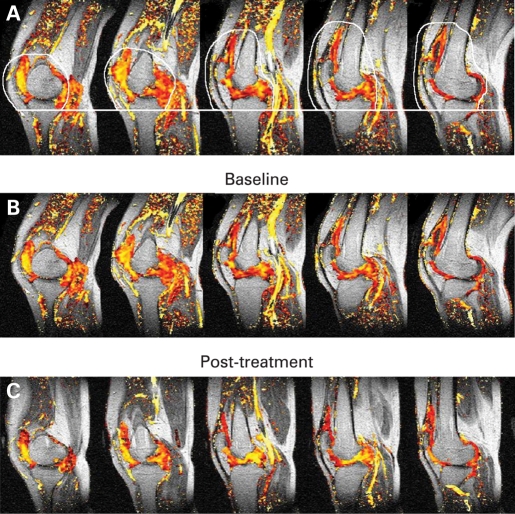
Five characteristic dynamic gadolinium-enhanced magnetic resonance imaging sagittal scans acquired from the knee with superimposed colour data showing values of initial rate of enhancement (IRE) across synovial space following gadolinium enhancement—pixels shown in yellow represent high IRE values whereas red show relatively lower values. Image (A) shows global region of interest outlined in white, which defines the extent of the synovitis at a level of 10 pixels below the tibial plateau to the top of the suprapatellar pouch, avoiding enhancement from muscle and arteries in each of the five sagittal images. Images (B) and (C) are an example of pre and posttreatment, respectively.
